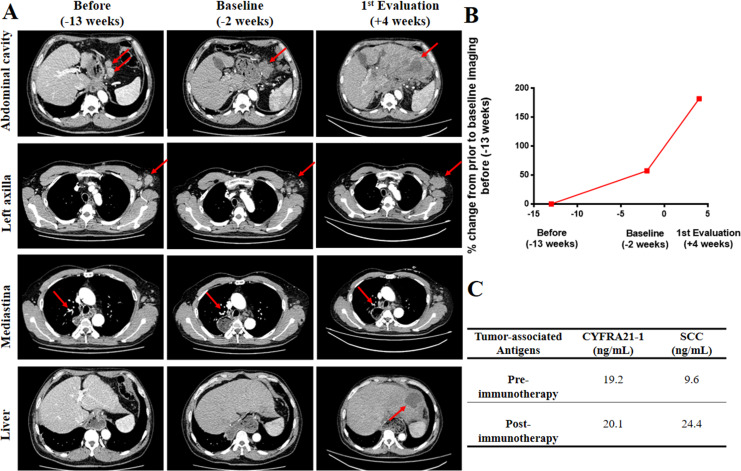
Case study of a patient in his mid-40s with HPD during immunotherapy. (A) CT scans were performed 13 weeks before starting anti-PD-1 treatment (column 1), at baseline (2 weeks before starting immunotherapy, column 2), and at first evaluation (4 weeks after starting immunotherapy, column 3). CT scans from lines 1 to 4 revealed the changes in lymph nodes in the abdominal cavity, left axilla and mediastina, respectively. New liver lesion appeared. The red arrows indicate tumer lesions.(B) Rate of change in growth pattern in the patient, who developed HPD to camrelizumab. Compared with the tumor image (−13 weeks), the tumor lesions at baseline (−2 weeks) and at first evaluation (4 weeks after starting immunotherapy) showed approximately 57% and 181% increases (79% increase compared with baseline imaging), respectively; 2.5-fold increase in progressive pace compared with preimmunotherapy. (C) Changes in tumor-associated antigens before and after immunotherapy. CYFRA21-1, cytokeratin-19 fragment; HPD, hyperprogressive disease; PD-1, programmed cell death 1; SCC, squamous cell carcinoma.
